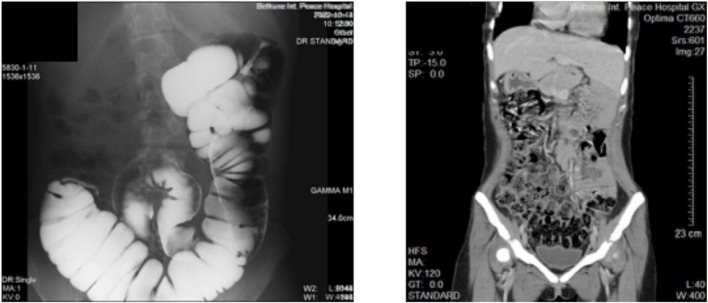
Imaging images of the patient’s abdomen. (Left) Barium examination. (Right) Full abdominal CT scan.
